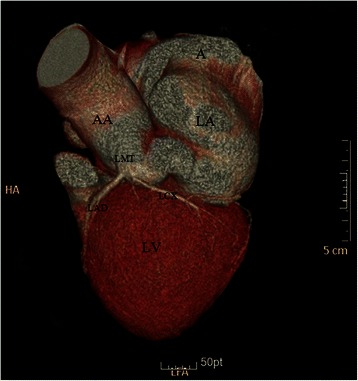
3D-reconstruction of coronary CT demonstrated the positional relation of the coronary aneurysm and surrounding structures. Abbreviations LV: Left ventricle; LA: Left Atrium; A: Aneurysm; AA: Ascending Aorta; LMT: Left Main Trunk; LAD: Left Anterior Descending; LCX: left circumflex coronary artery
